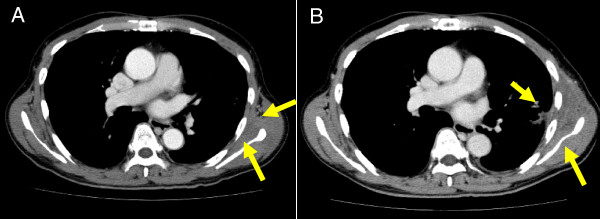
A 64-year-old man with adenocarcinoma after SRT. A) At 3 months after completion of SRT, contrast-enhanced CT shows suspicious findings in the left chest wall, such as slight asymmetry and indistinct intermuscular fat planes (arrows). B) At 15 months after SRT, contrast-enhanced CT shows definitive chest wall edema, evidenced by swelling of the left chest wall with an area of low attenuation (arrows). No rib fracture was seen at this time.
